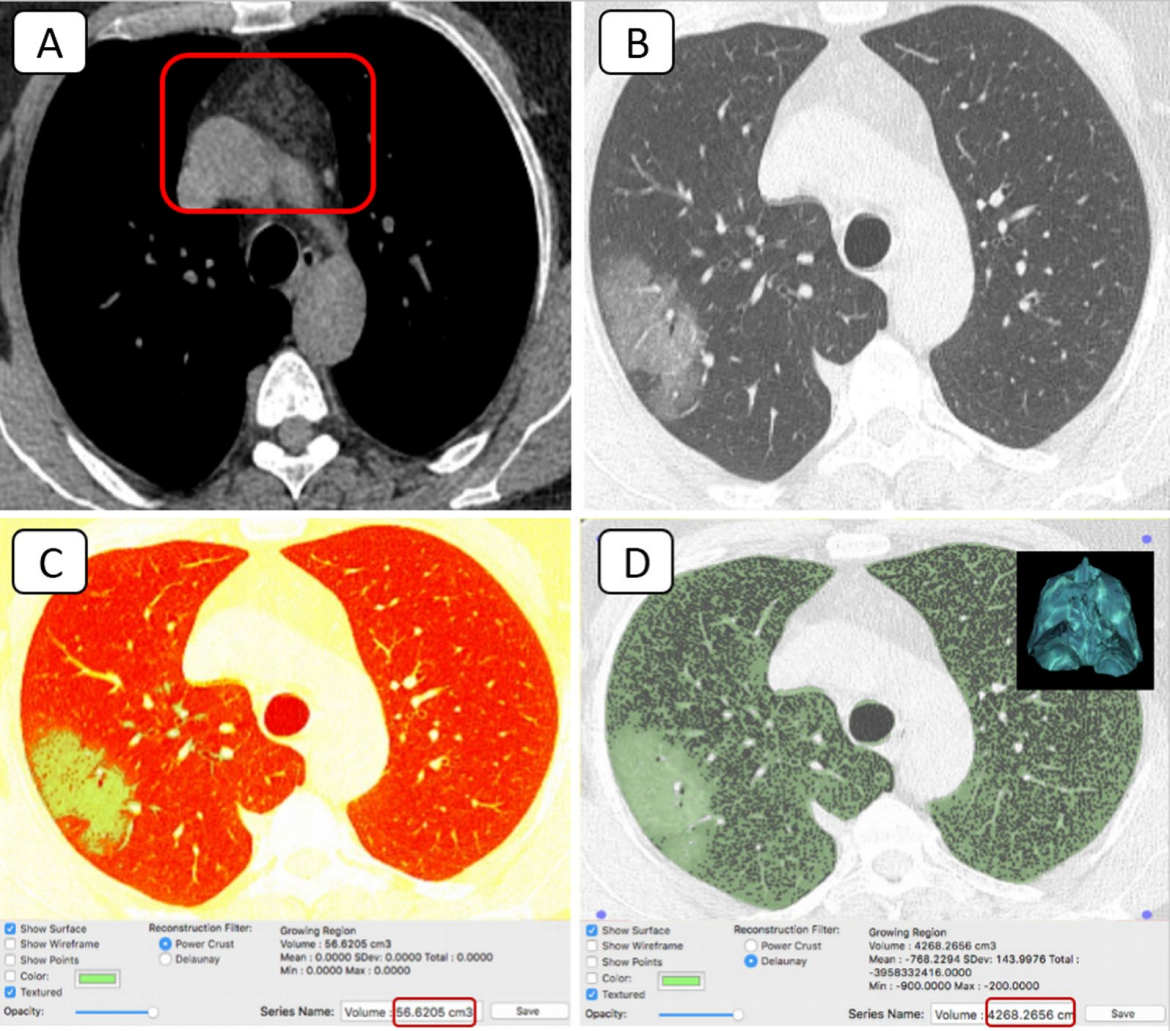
A 48-year-old male COVID-19 patient complained of fever, dry cough, and chest pain: A Axial mediastinal window CT showed nodular infiltration of the thymic gland (grade 2) [red square]. B Axial lung window CT showed a right upper lobar sub-pleural ground-glass patch. C–D Axial 3D volumetry cuts highlighted the right sub-pleural ground-glass patch. The pathological lung volume was around 1.3% of the total lung volume
